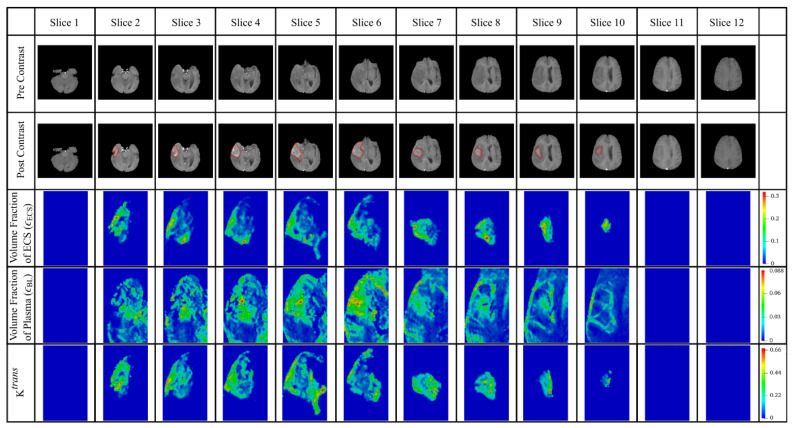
Tissue heterogeneous properties extracted from patient DCE-MR images. The interface between the brain tumor and its holding tissue is marked in red in the post-contrast images.
